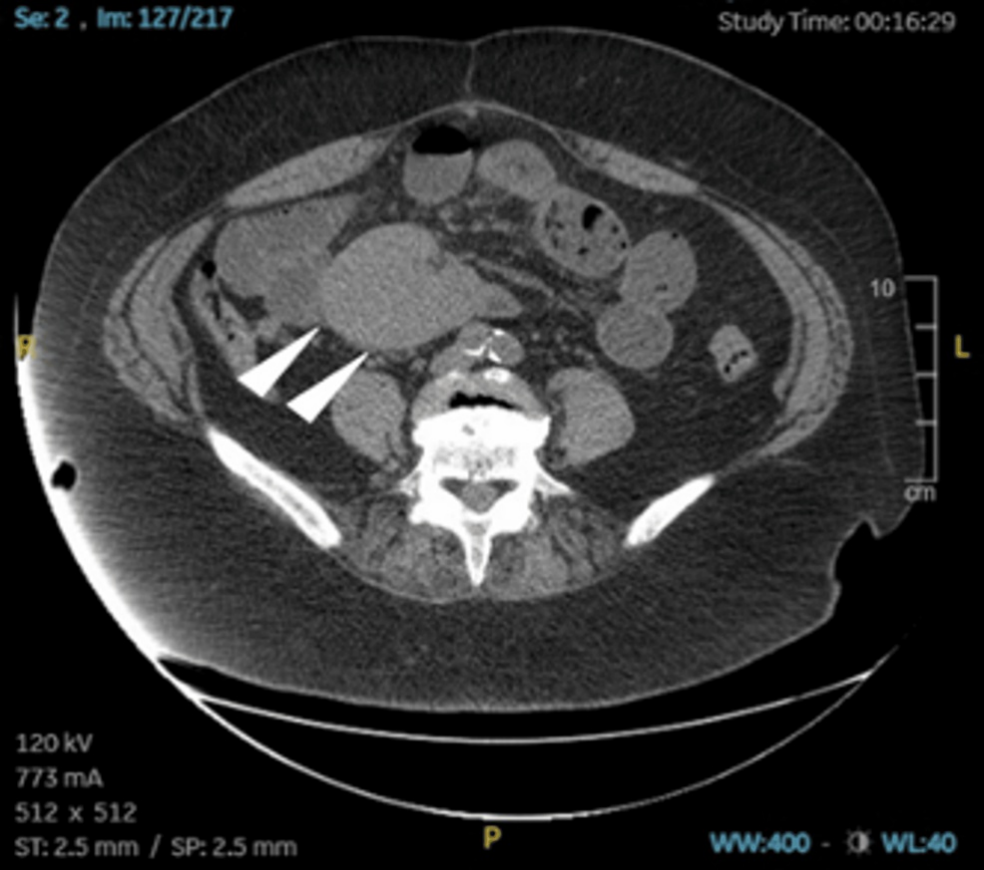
CT of the abdomen and pelvis without contrast, supine position, axial view displaying distended loops of small bowel, and adhesions near the NET (arrowheads) taken at initial ER presentation. NET: neuroendocrine tumor
